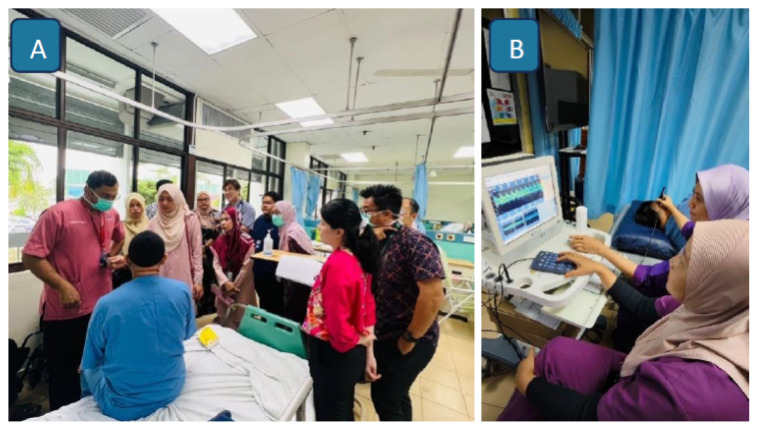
A) Stroke grand rounds are held twice weekly at the Acute Stroke Unit; B) Transcranial doppler ultrasound is used for screening intracranial vascular stenosis
